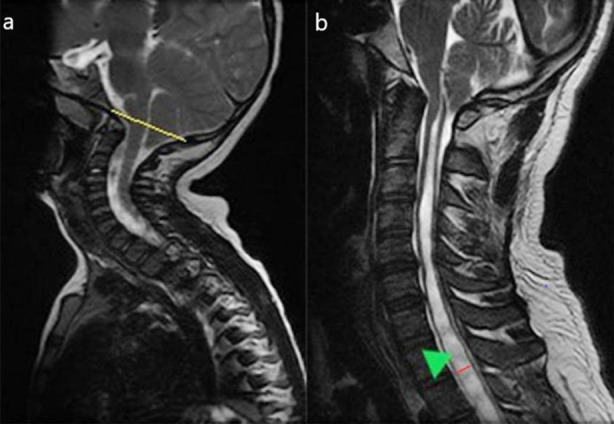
(a) Sagittal T2W sequence of MRI, showing tonsillar descent below the line drawn from basion to ophisthion (yellow line). (b) Sagittal T2W sequence of MRI, showing syrinx formation extending from craniocervical junction to dorsal spine level (green arrowhead). Red line depicting diameter of syrinx in anteroposterior dimension.
